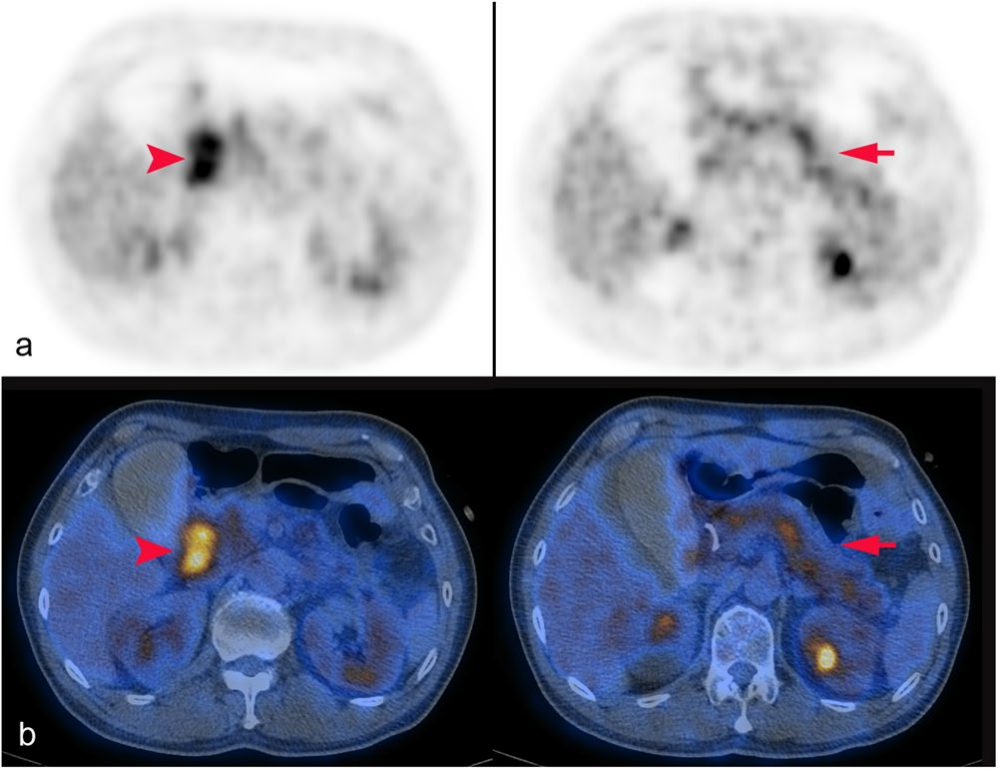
Pancreatic cancer. Axial PET (a) and PET/CT fused (b) images showed intense hypermetabolic areas from pancreatic head to tail (diffuse morphology, arrow), and the most intense focal area at periampullary region (arrowhead). No definite extrapancreatic lesion was found. The pancreatic tumor showed a SUVmax of 8.4, and a high gray-level zone emphasis value of 201.3. The patient underwent Whipple’s surgery confirming the diagnosis of pancreatic cancer. The disease was complicated with ischemic bowel disease and multi-organ failure developed, and the patient died despite intensive care support.
